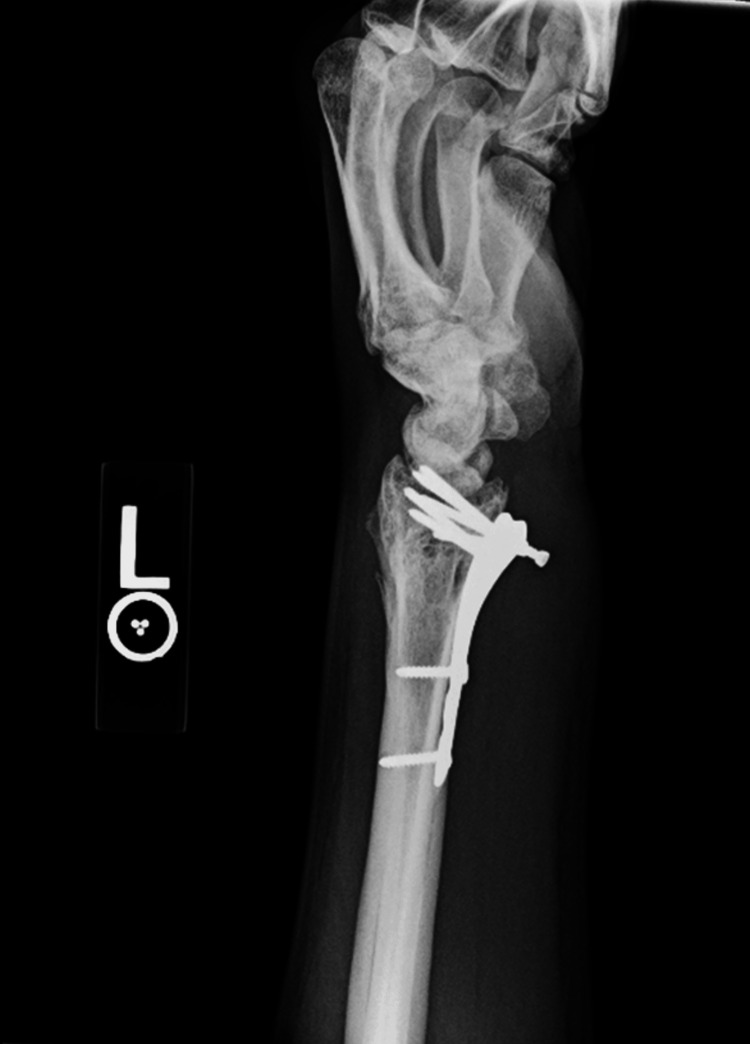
Left lateral wrist X-ray demonstrating volar screw displacement
